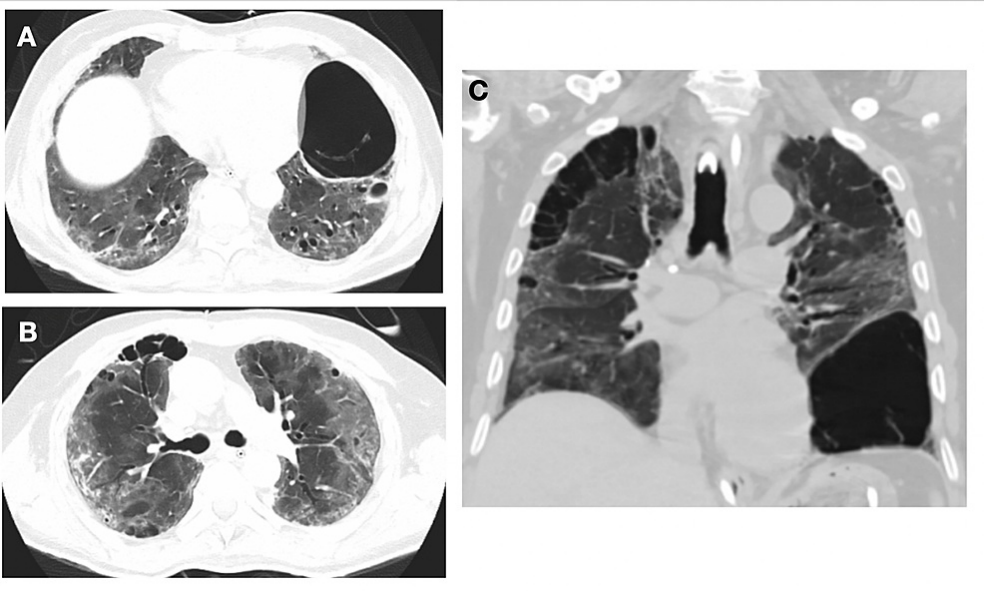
Pulmonary cystic changes associated with COVID-19. Chest CT coronal (A): Fibrotic appearance changes with increased density of the lung parenchyma and thickening of the central and peripheral interstitium, bronchial thickening, ground-glass opacities, and cystic lesions. Chest CT coronal (B): Cystic changes of paraseptal emphysema-like in the right upper lobe, ground-glass opacities, and bronchial thickening. Chest CT sagittal (C). Large left basal cyst measuring 70 x 84 mm. Cystic changes in right upper lobe bronchial thickening and ground-glass opacities.
